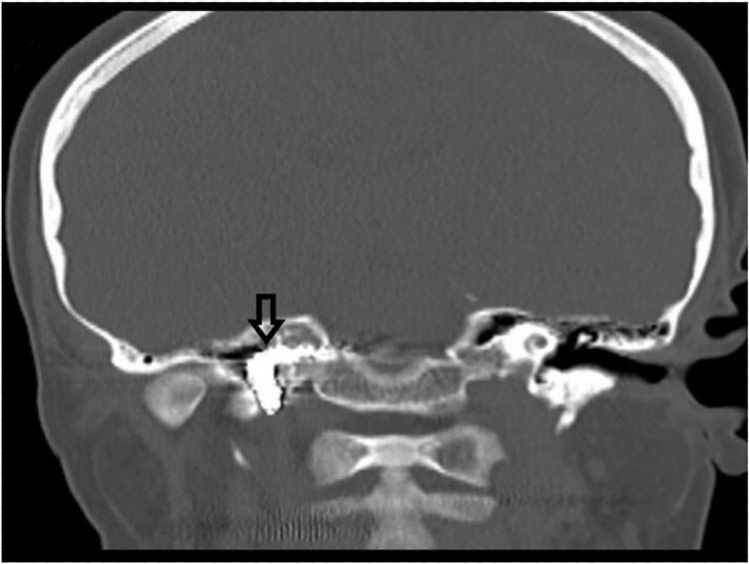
CT scan, showing complete occlusion of the right ICA at the petrous and cervical parts (arrow).
